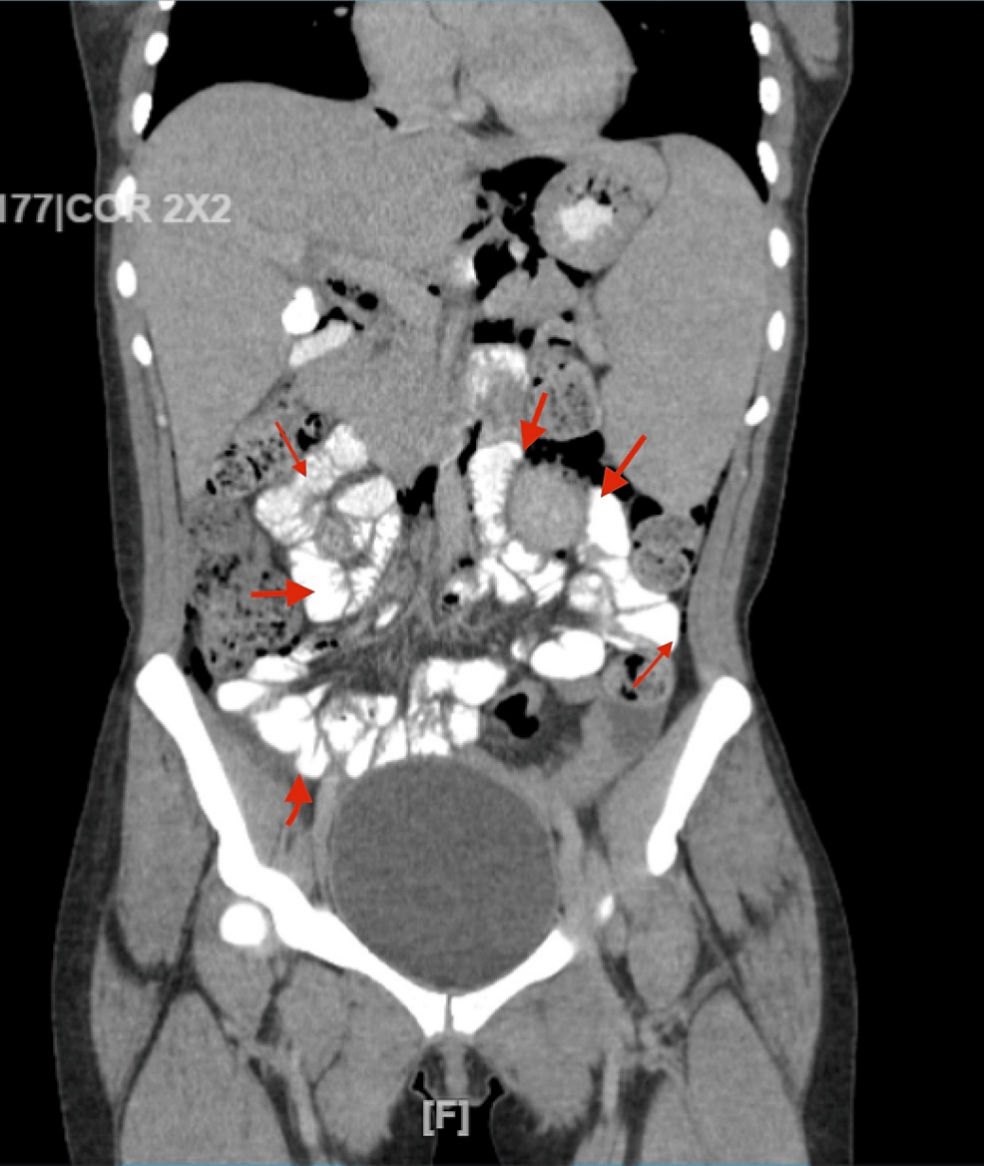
CT of the abdomen with oral contrast showing no intraperitoneal or retroperitonal contrast extravasation. The red arrows show contrast within the stomach, duodenum as well as bowels.
